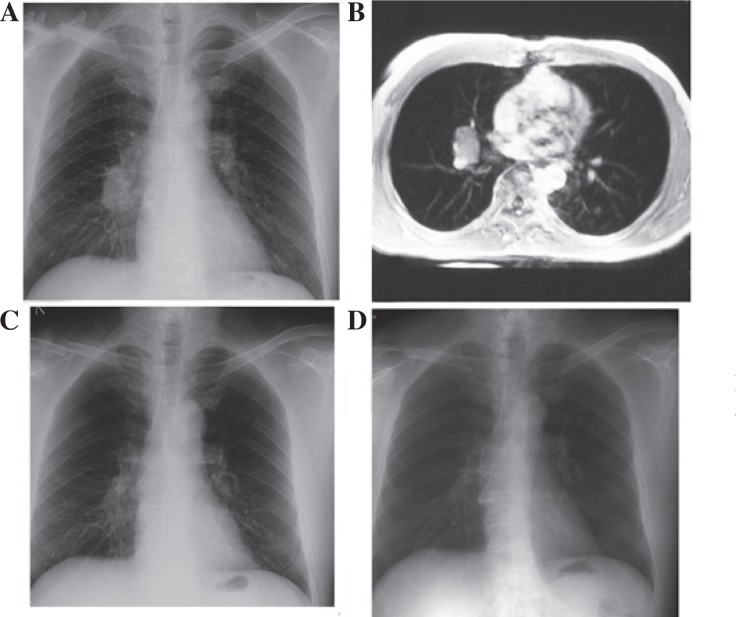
Chest radiograph and MRI of the lungs. (A) Chest radiograph identifiying a lesion in the right hilus pulmonis with a clear edge (November 26, 2002). (B) MRI identifying a 3×2-cm lobulated node in the right hilum (November 26, 2002). (C) Chest radiograph detecting no mass, but indicating the presence of a chaotic blood vessel system (December 29, 2002). (D) Normal chest radiograph (December 20, 2012).
